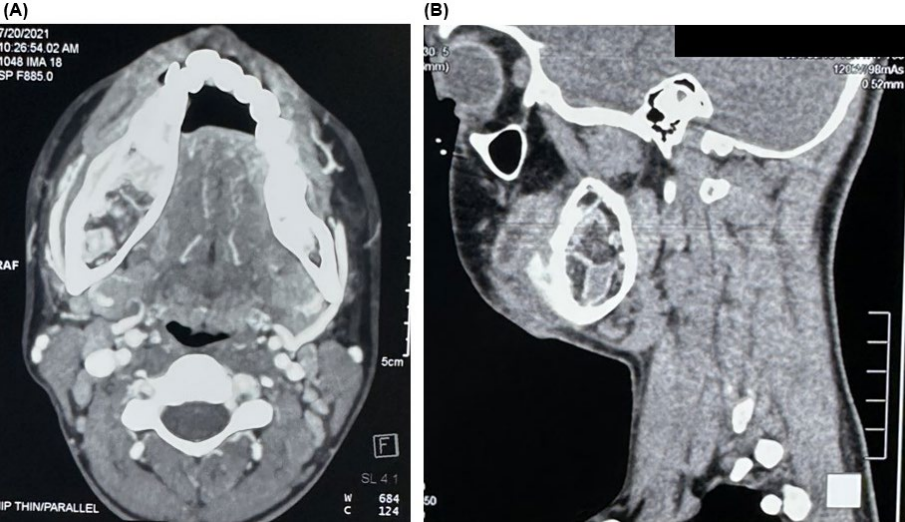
Computed tomography angiogram of mandible shows lytic expansion of body of right mandible and dilated right mandibular canal. (A): Axial view, (B): Sagittal view.
